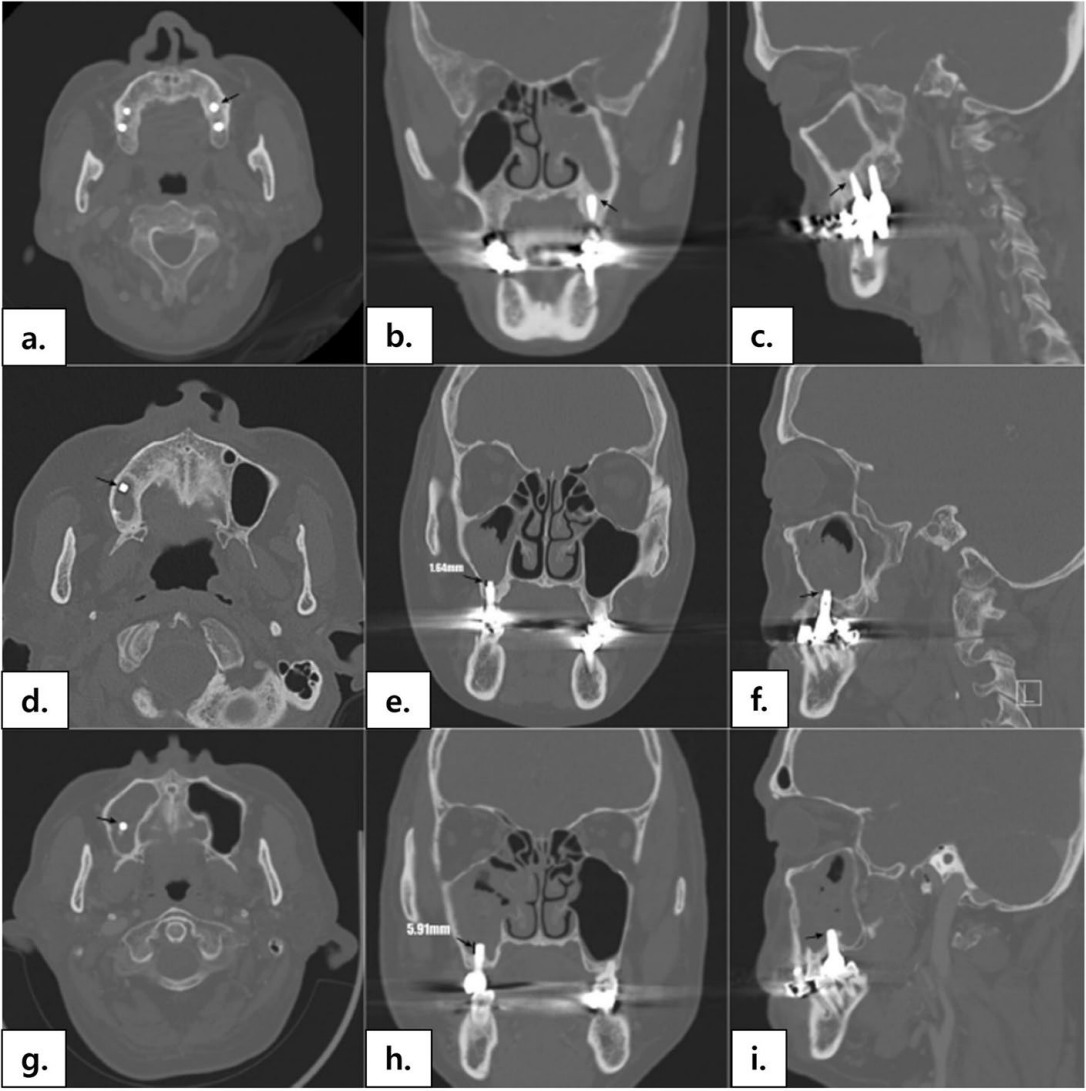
Evaluation of dental implants on paranasal computed tomography: screw part of dental implants are seen as high density materials, some of which penetrates the maxillary sinus floor (black arrows). Grade 1 (a–c): dental implant does not penetrate the maxillary sinus floor; Grade 2 (d–f): the implant penetrates the maxillary sinus floor but does not 3 mm; Grade 3 (g–i): the length of penetration into the maxillary sinus exceeds 3 mm.
